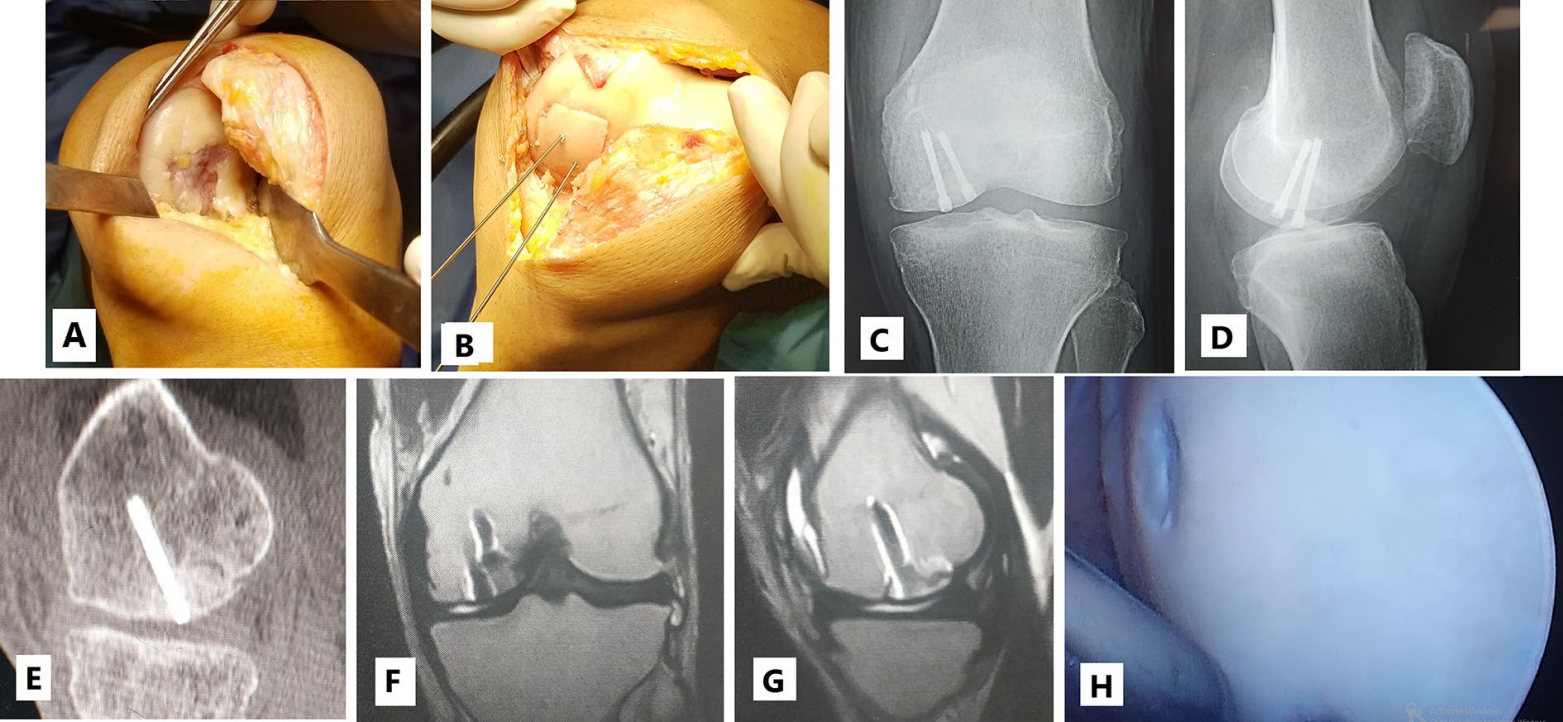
45 years old male presented 3 weeks after left knee trauma. A: Intraoperative image showing uncontained defect in the medial femoral condyle measuring 32 mm * 19 mm. B: Shell osteochondral allograft fixed by 2 headless compression screws. C and D: 3 months postoperative X-ray showing well contoured graft incorporation. E: 5 months postoperative CT scan showing well contoured graft incorporation. F and G: 5 months postoperative MRI showing 75–100% cartilage fill with good contour. H: 5 months postoperative second look arthroscopy showing intact hyaline cartilage
